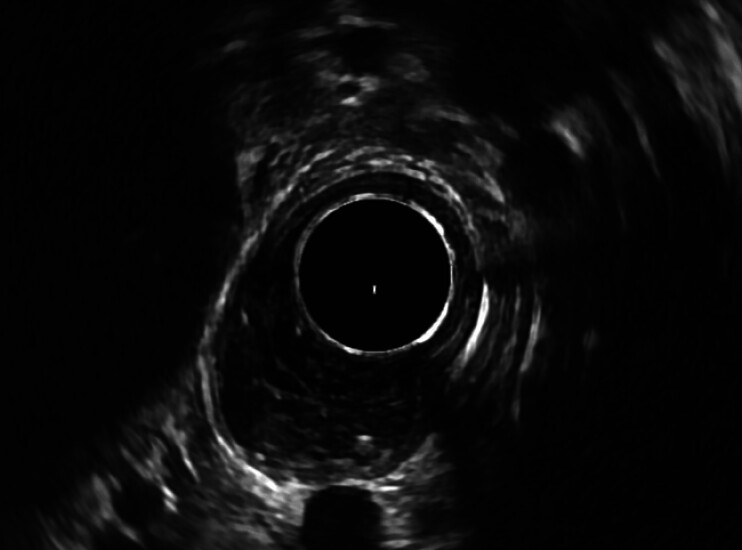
Endoscopic ultrasound confirmed an inhomogeneous hypoechoic and well-demarcated mass localized in the submucosa.
